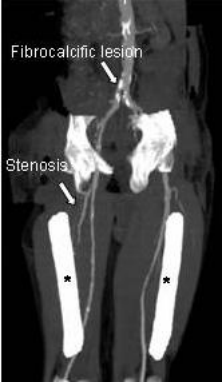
Abdominal computed tomography angiography of our patient's aorta and lower limbs. Widespread fibrocalcific atherosclerotic lesions are present. Both renal arteries and iliac branches are affected by severe stenosis (70% to 85%). Tibial arteries are occluded, thus preventing the examination of parts of the legs.
